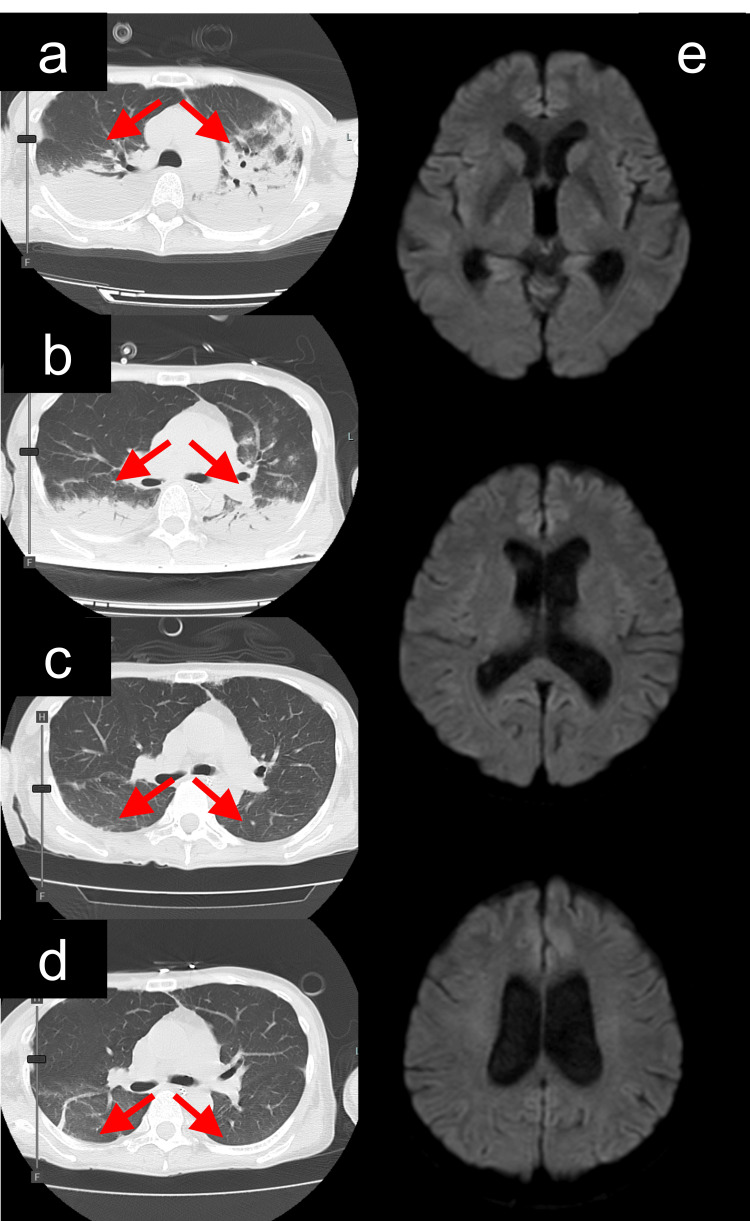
Serial studies demonstrating resolution of pulmonary edema (red arrow) and prevention of vasospasm despite following clazosentan administration a) Chest computed tomography (CT) performed immediately after coil embolization demonstrated still pulmonary edema (red arrow). b) Chest CT obtained on postoperative day (POD) 4 showed a reduction in pulmonary edema (red arrow). c) Chest CT on POD 7, corresponding to day 4 of clazosentan administration, demonstrated resolution of pulmonary edema (red arrow). d) Chest CT on POD 11, also corresponding to day 8 of clazosentan administration, confirmed sustained resolution of pulmonary edema (red arrow). e) Brain magnetic resonance imaging (MRI) performed on POD 11 revealed no evidence of delayed cerebral ischemia (DCI).
